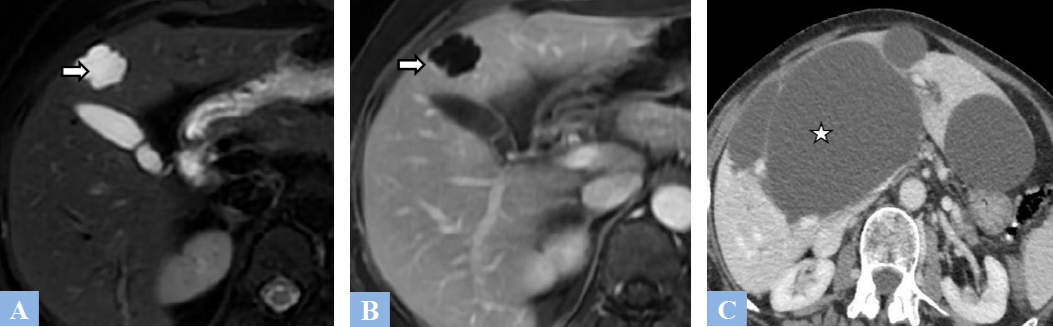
Examples of simple biliary cysts. A. T2-weighted fat-suppressed MR image in the axial plane showing a biliary cyst appears as a T2 hyperintense lesion with lobulated contour (arrow). B. T1-weighted fat-suppressed MR image in the axial plane after gadolinium administration at the portal phase of enhancement. No wall enhancement observed (arrow). C. CT image in the axial plane at portal phase of enhancement showing well-defined hypodense cystic lesions (asterisk) without walls, located in segment IV and the left lobe.
